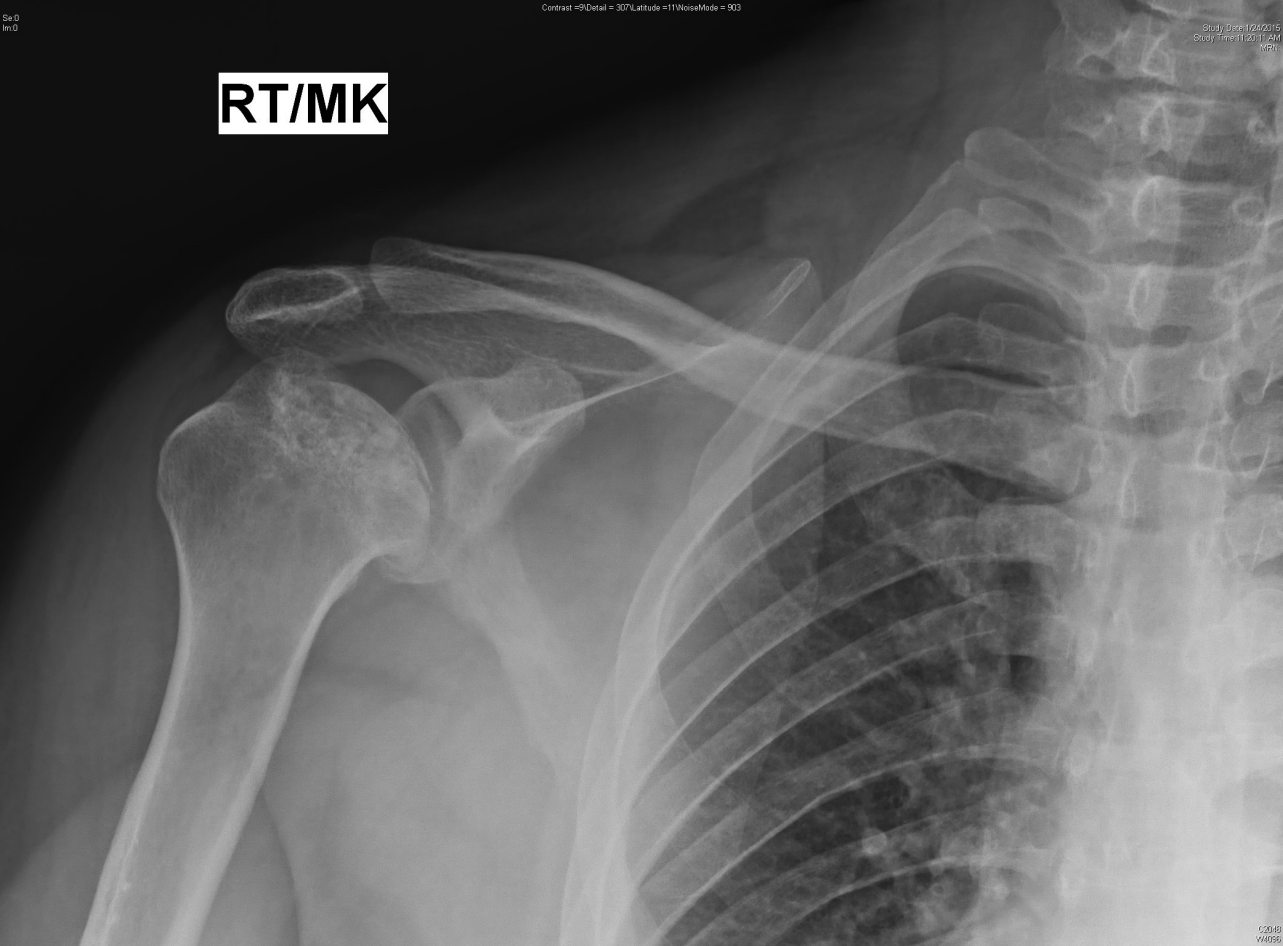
X-ray right shoulder anteroposterior view showing avascular necrosis of the head of the humerus
